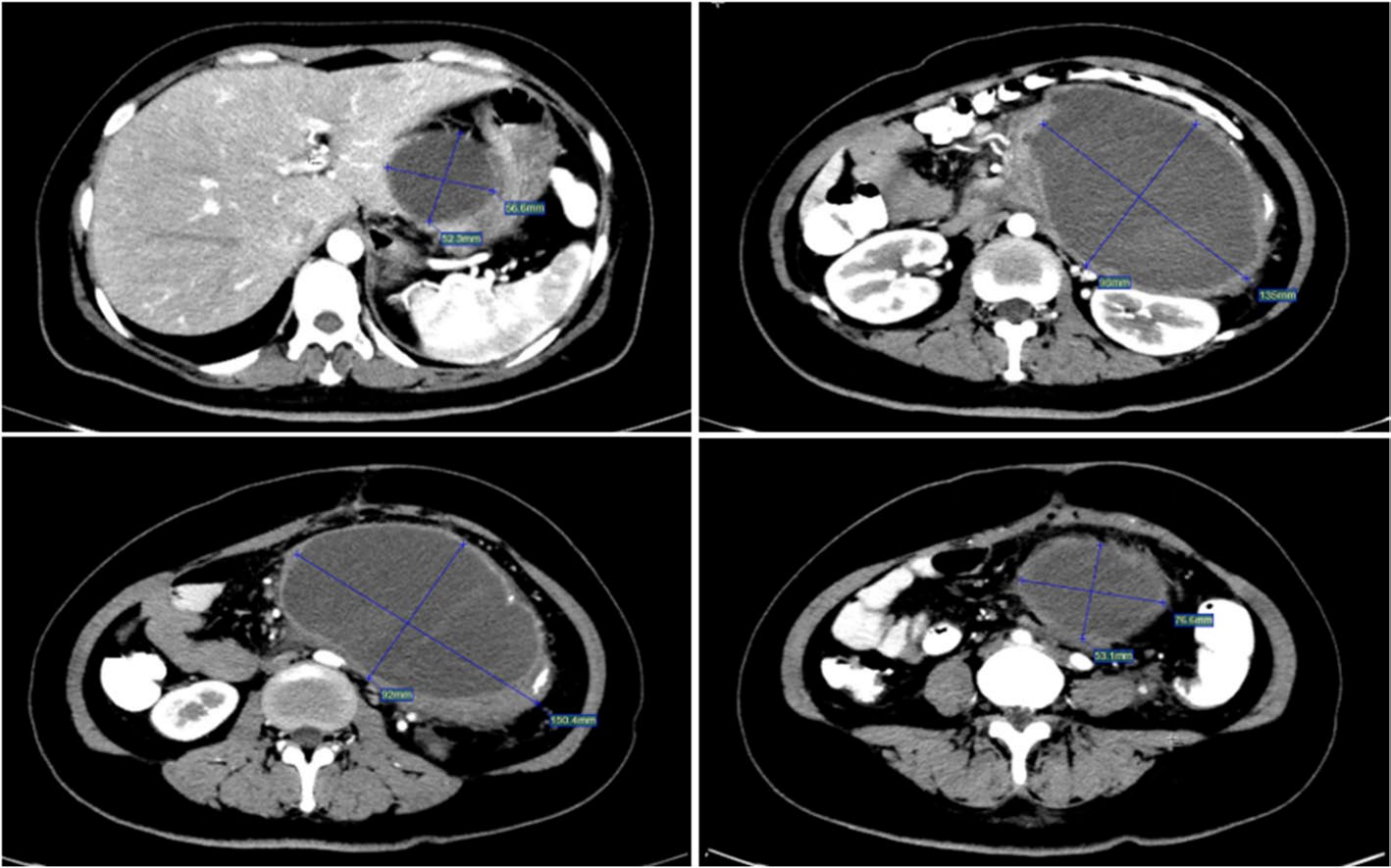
The density of the RPH showed a significant reduction. Maximum cross-sectional area is approximately 13.5 cm × 9.8 cm. Average CT value: 19Hu
